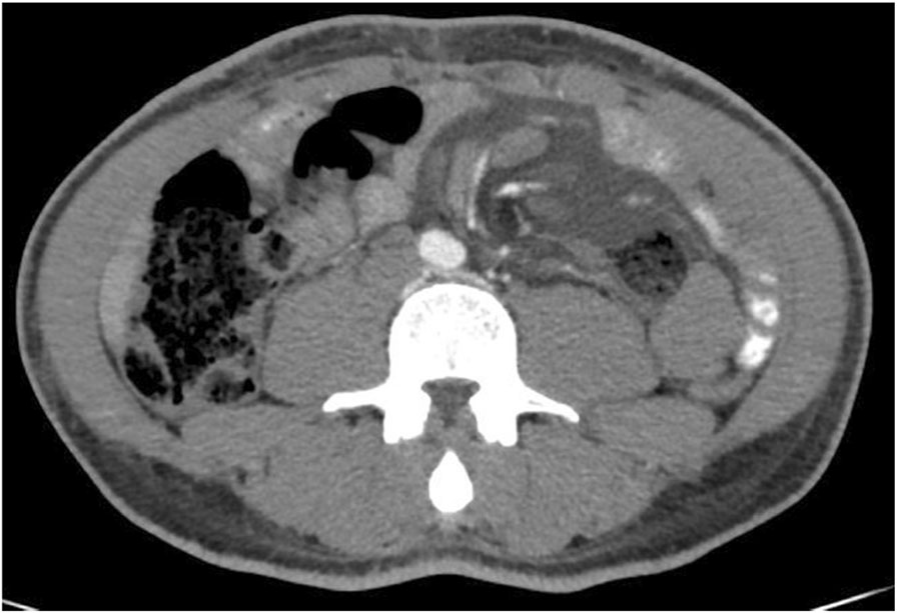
Abdominal computed tomography with intravenous contrast showing diffuse enlargement of mesenteric lymph nodes
